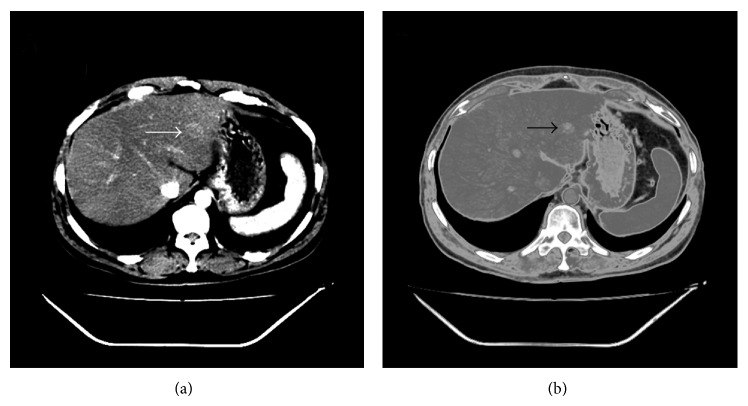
CT showed one lesion in the left lobe liver of case 2 (arrows). (a) contrast enhancement imaging; (b) delayed imaging.
